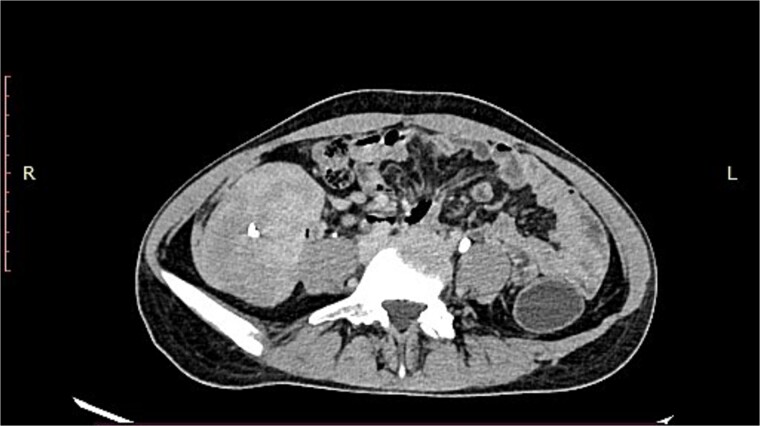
CT image showing a well-defined smooth lobulated heterogeneously enhancing mass lesion in the right paracolic gutter and right iliac fossa, with calcific focus within.
